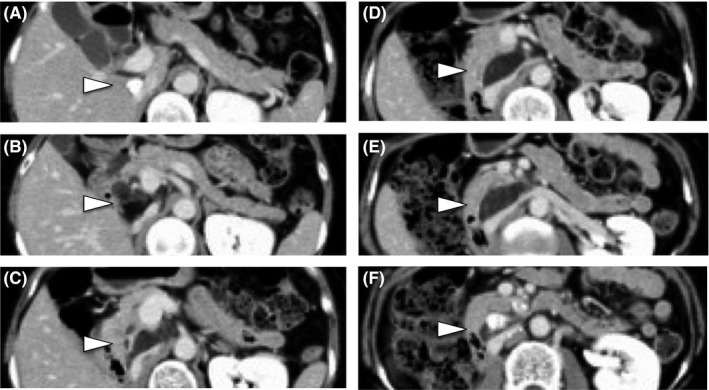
Preoperative computed tomography images. (A‐F) There is a low‐density mass including calcification around the inferior vena cava behind the liver and the head of the pancreas. Arrowheads in A‐F in figure 1 are pointing to a low density mass including calcification
